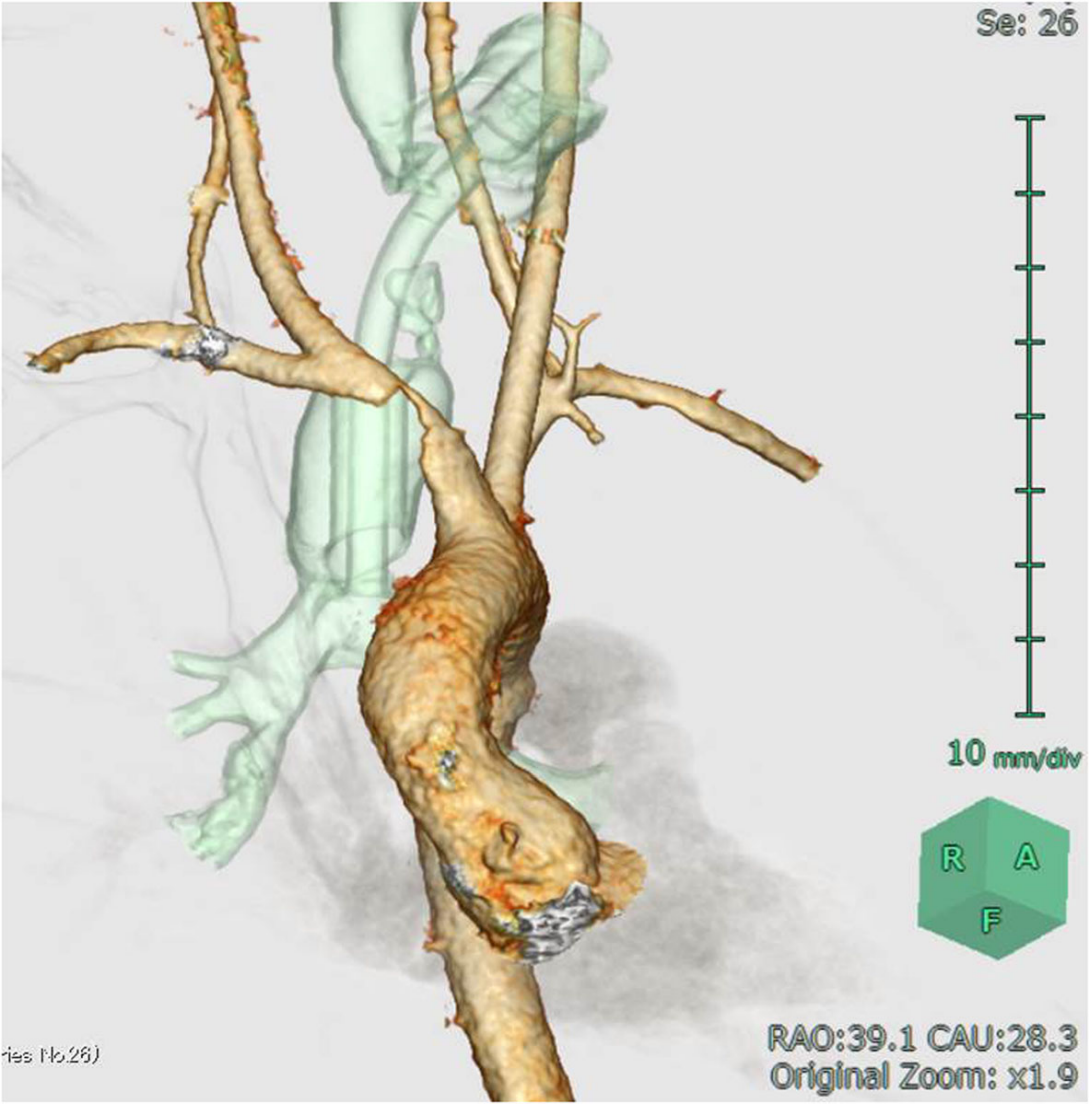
Pre-operative CECT in Case 2. Pre-operative CECT revealed that the innominate artery ran close to the anterior surface of the trachea. The part of the wall is irregular; therefore, it appeared to form a fistula in the site (CECT: contrast-enhanced computed tomography)
